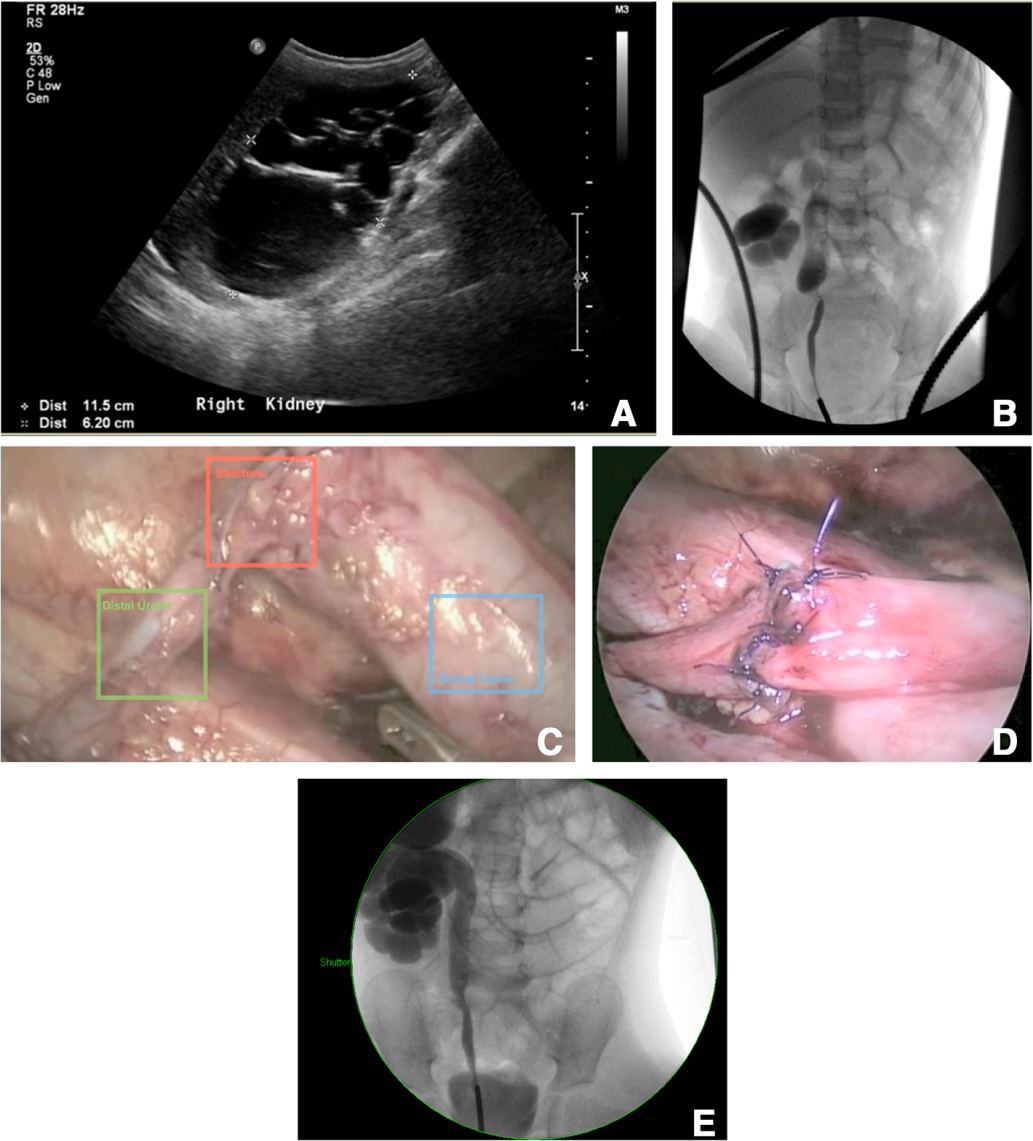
(a) Preoperative ultrasound showed SFU grade 4 hydronephrosis. (b) Retrograde pyelogram demonstrated right ureteral stricture at the level of the right mid-sacroiliac joint with proximal mega-ureter. (c) Laparoscopic view of the mid-ureteral stricture. (d) Image obtained at the end of the laparoscopic ureteroureterostomy. (e) Retrograde pyelogram showed a patent ureteral lumen after double-J stent removal
